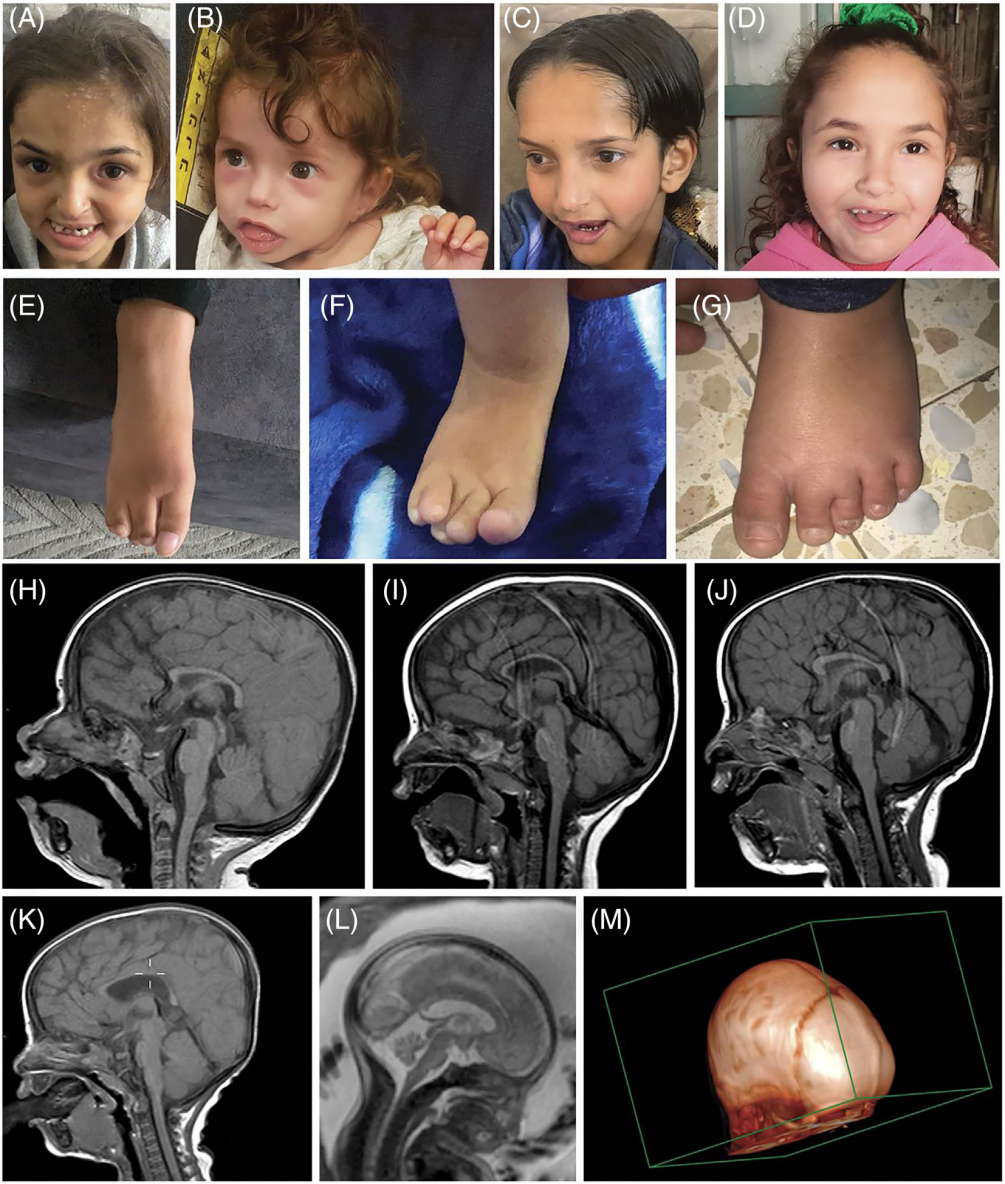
Clinical characterization. (A–D) Patients V:5, V:6, IV:4, and V:1, respectively. Craniofacial dysmorphism was apparent with normal to borderline small head circumference, characteristic triangular face with prominent forehead, slightly posterior‐rotated low‐set ears, and small chin. (E–G) Patients V:5, V:6, and V:1, respectively: over‐riding toes, with clinodactyly or syndactyly. (H–K) Brain MRI of patients IV:4, V:4, V:5, and V:9, respectively. (L) Brain MRI of patient V:6 in‐utero. All affected individuals showed thinning of corpus callosum with paucity of white matter. (M) Metopic stenosis of patient V:9. [Colour figure can be viewed at wileyonlinelibrary.com]
